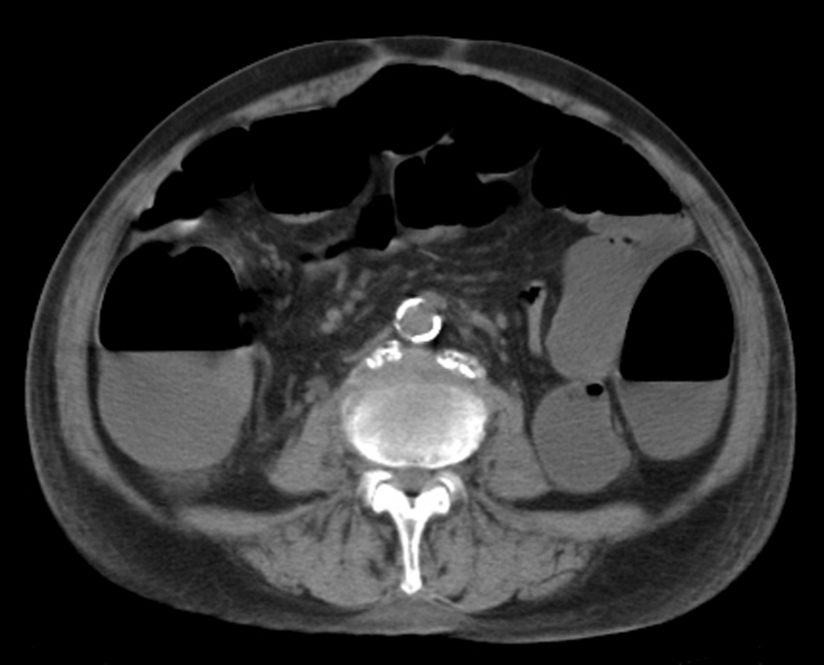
Abdominal computed tomography shows massive large bowel dilatation of the entire colon.
